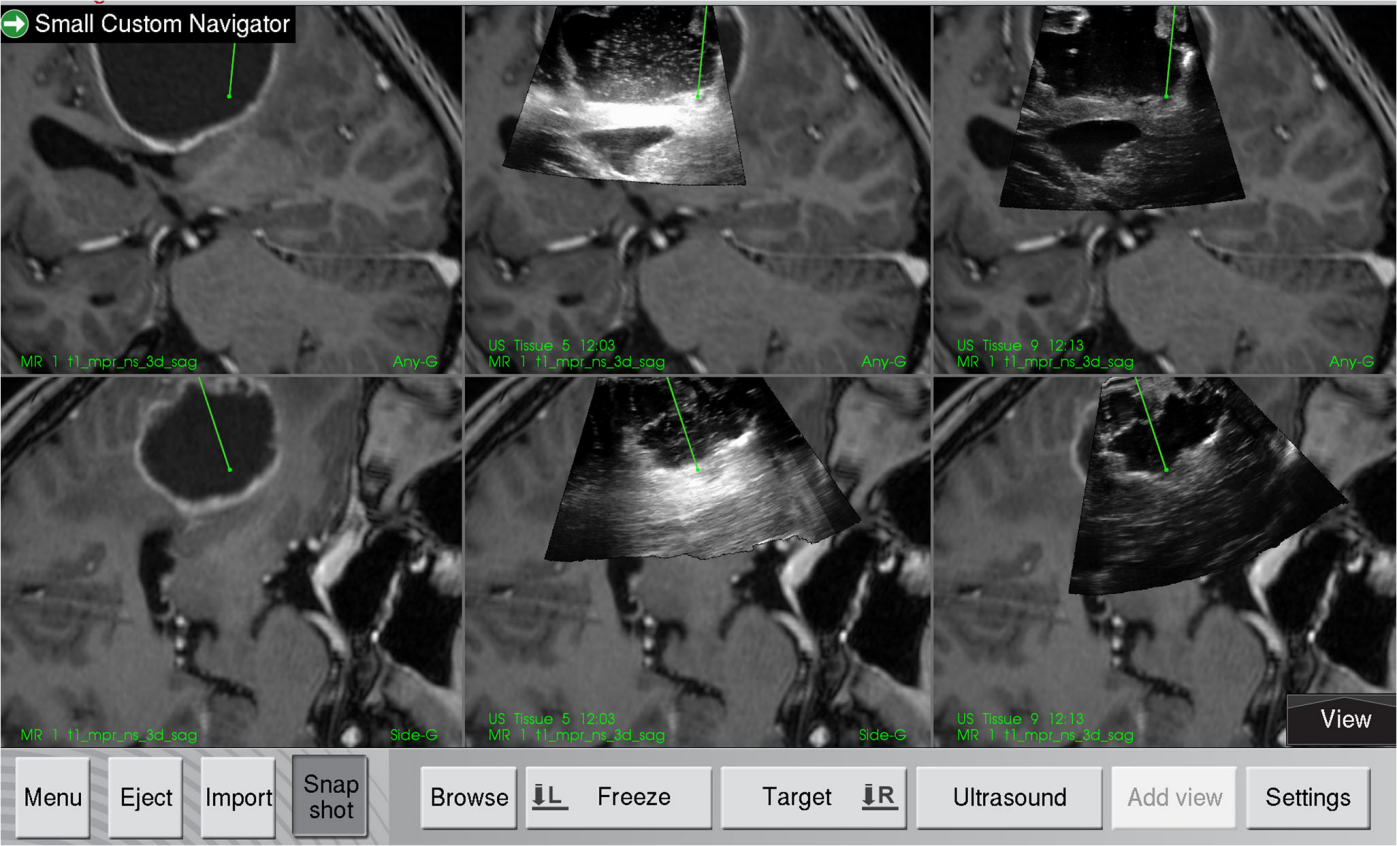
Display from the navigation system, showing two perpendicular image slices extracted from the MRI or 3D ultrasound volume according to the orientation of the navigated tool. The preoperative MR T1-weighted contrast image is shown to the left. The middle column shows the ultrasound images acquired with Ringer’s solution in the resection cavity, and in the right column, the ultrasound obtained with ACF-3 is shown overlaid the MR images
